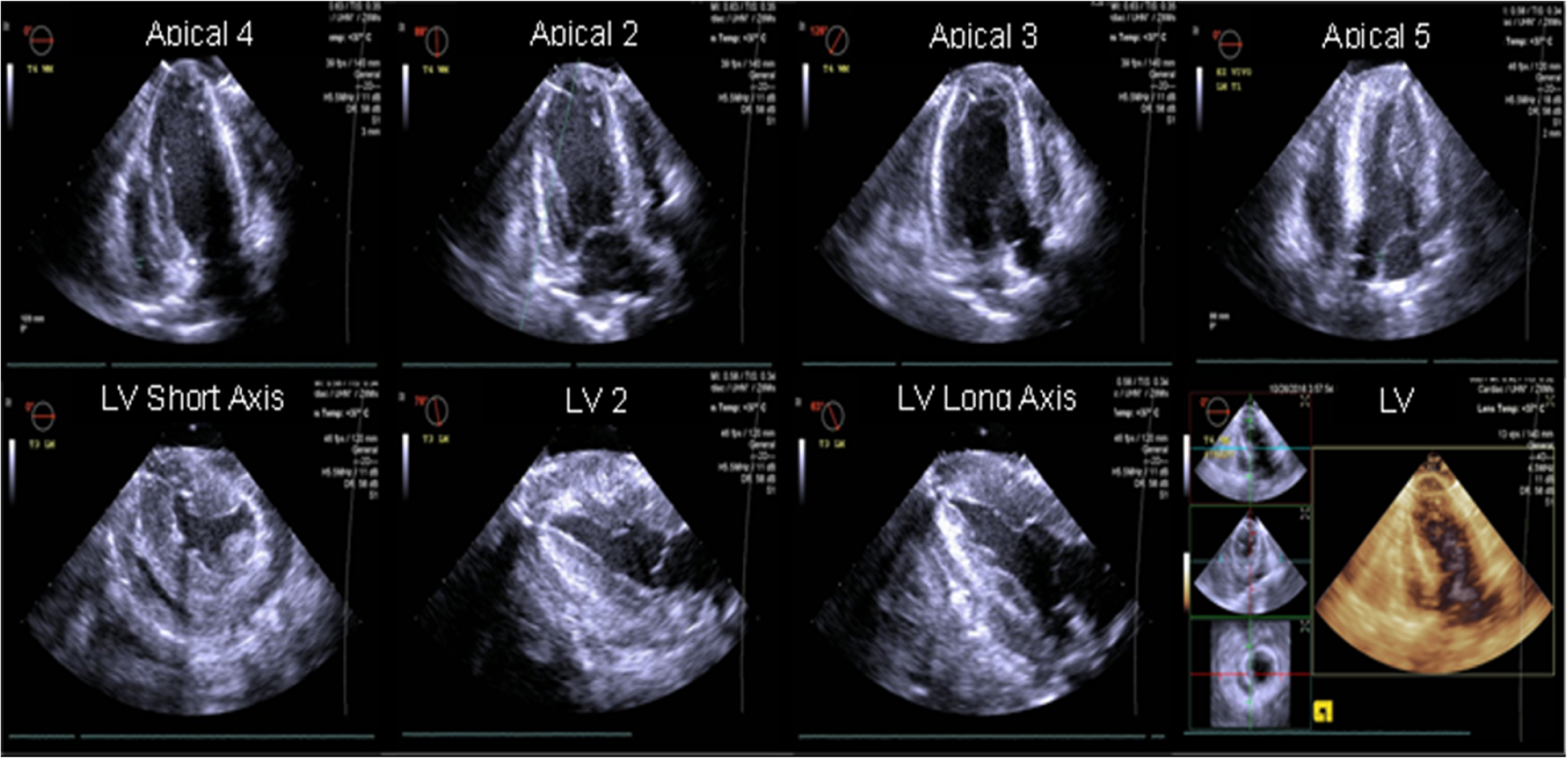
Representative echocardiographic images obtain with our 3D-printed set-up in working mode during ESHP. ESHP, ex situ heart perfusion; LV, left ventricular
